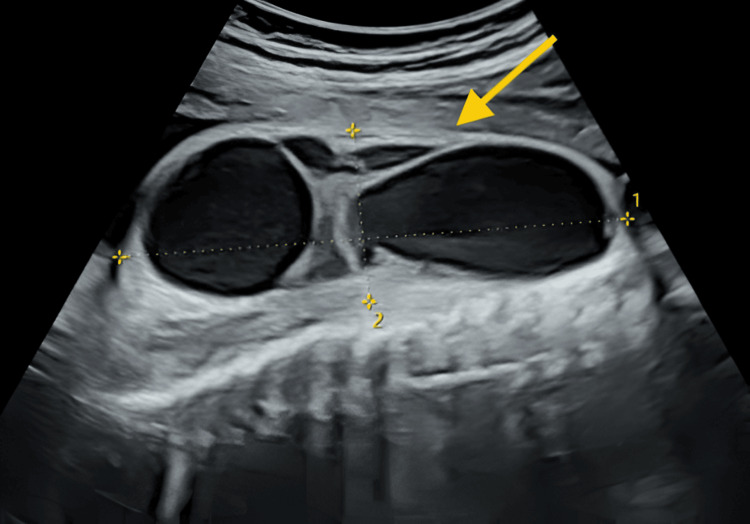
Fetal ultrasound image of the posterior subcutaneous cystic mass measuring 98 × 76 × 33 mm at 33 weeks of gestation
